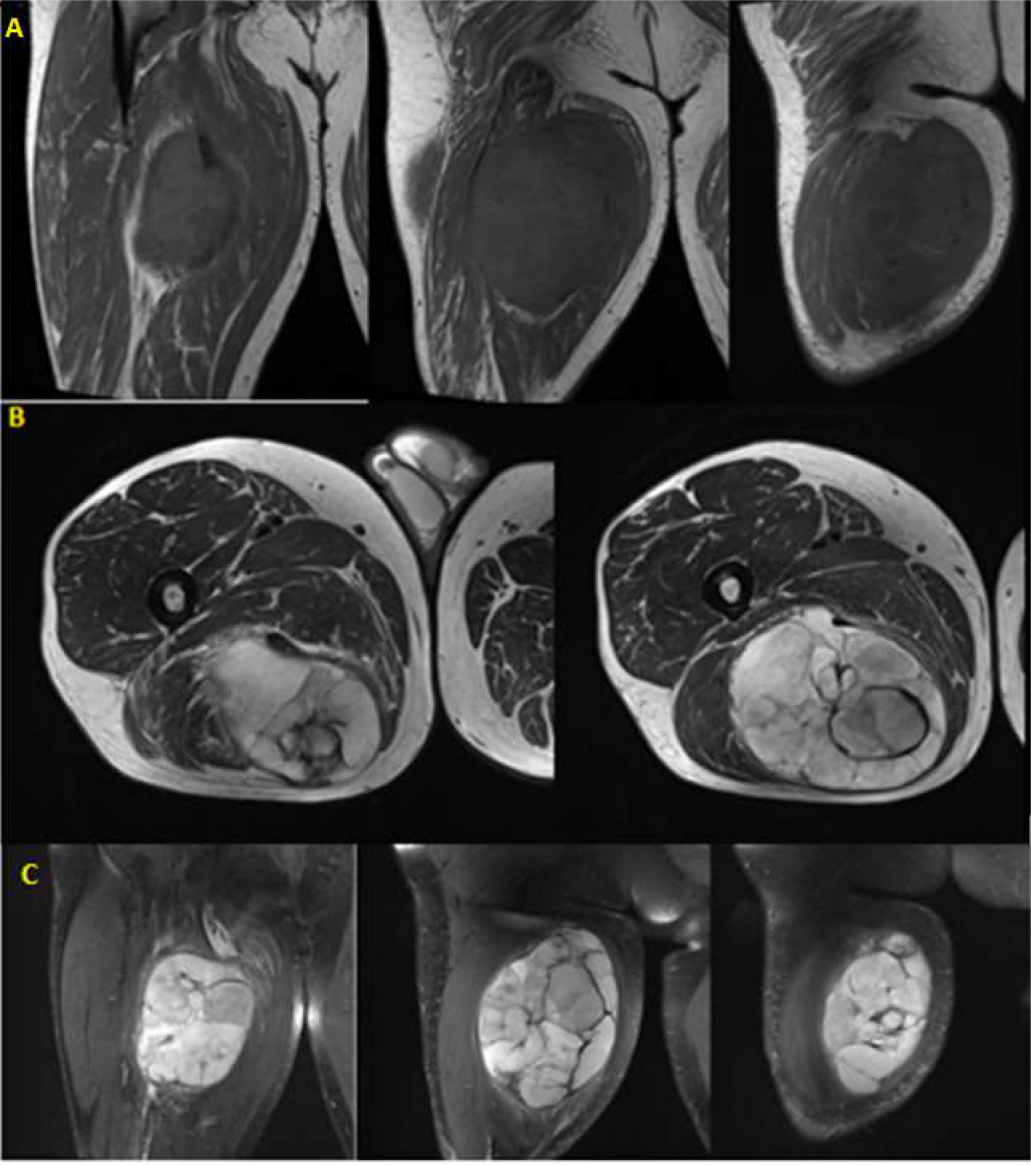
MRI of the posterior region of the right femoral region at different levels of the lesion, in diverse sections (A) coronal plane in T1 (isointense signal); (B) axial plane in T2 (hyperintense signal); (C) coronal plane in pd (proton density) where it appears hyperintense too.
